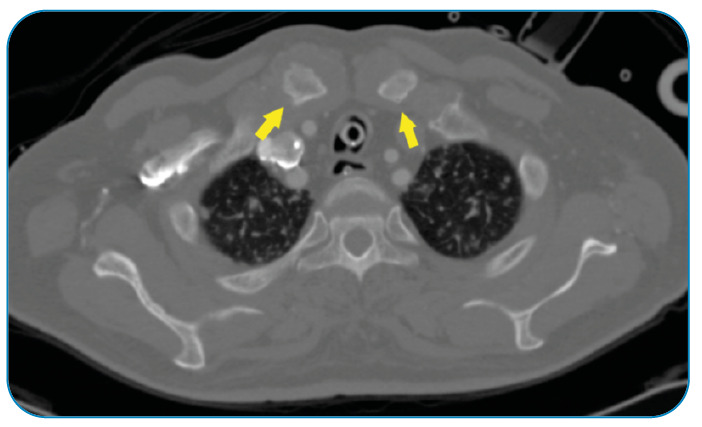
Computed tomography of the thorax showing bilateral sternoclavicular joint abscesses.
